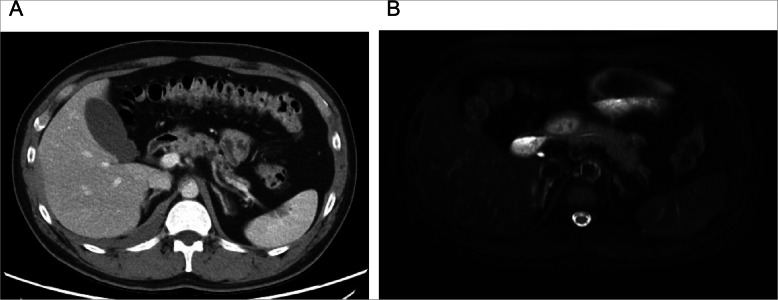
a Abdominal CT showing multiple small hypodense lesions in the head and body of the pancreas. b Magnetic resonance imaging demonstrates a normal pancreas
